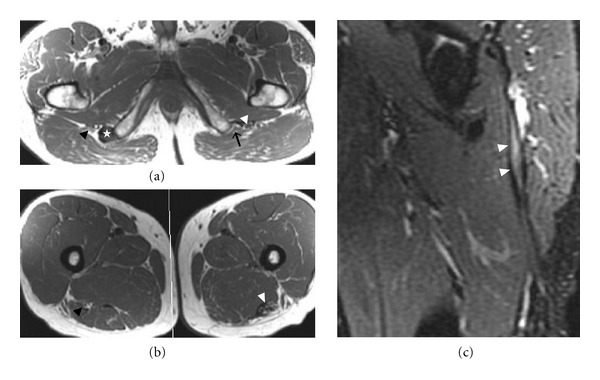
Extensive tear of the left hamstring muscle origin with sciatic nerve scarring in a 54-year-old patient after a water skiing injury. Axial T1-weighted images (a) and (b) identify a tear of left hamstring at the level of origin (black arrow in (a)). There is thickening and abnormal signal of the adjacent sciatic nerve (white arrowheads) extending distally (b), consistent with secondary entrapment from scarring. This finding is also demonstrated on a sagittal fat-saturated T2-weighted image (c). On the right side, the right sciatic nerve (black arrowheads) has normal caliber and signal intensity, and an intact hamstring muscle origin is present (star).
